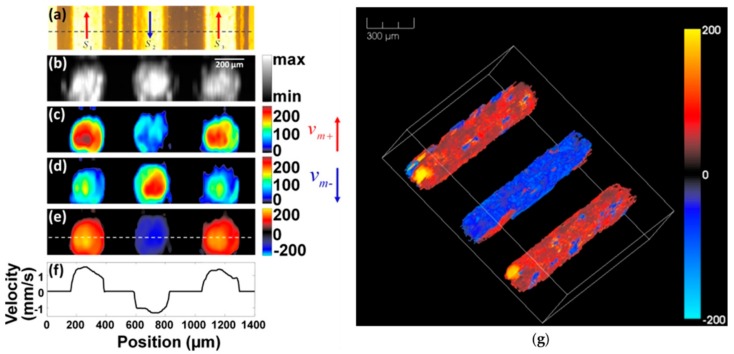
PA Doppler flowmetry. (a) PA Doppler bandwidth as a function of flow velocity; (b–f) PA flow imaging at different velocities and directions; (g) a 3D visualization of the PA flow imaging. Reprinted with permission from [101].
